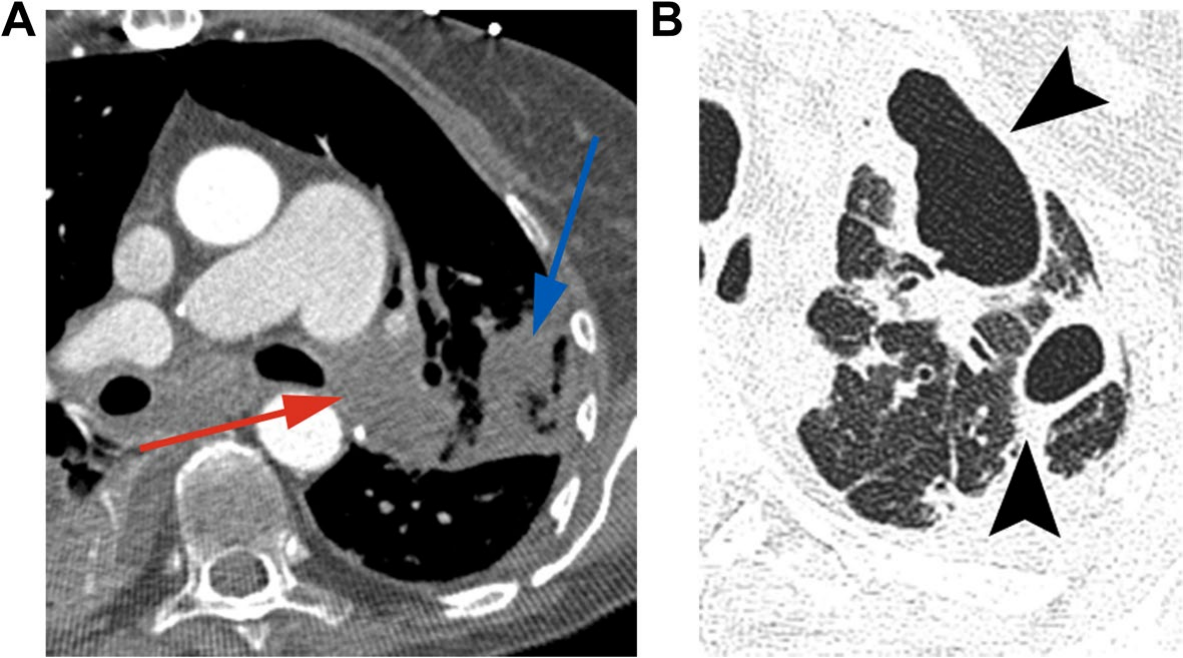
Pulmonary embolism and infarct in a 59-year-old female one year out from lung transplants for fibrotic hypersensitivity pneumonitis. A Axial contrast-enhanced CT image timed for the pulmonary arteries shows a large filling defect occluding the left pulmonary artery (red arrow). An associated subpleural consolidation represents pulmonary infarction (blue arrow). B Followup axial CT image (lung window) shows evolution of pulmonary infarct to cavitations most pronounced in the left lung apex (arrowheads)
